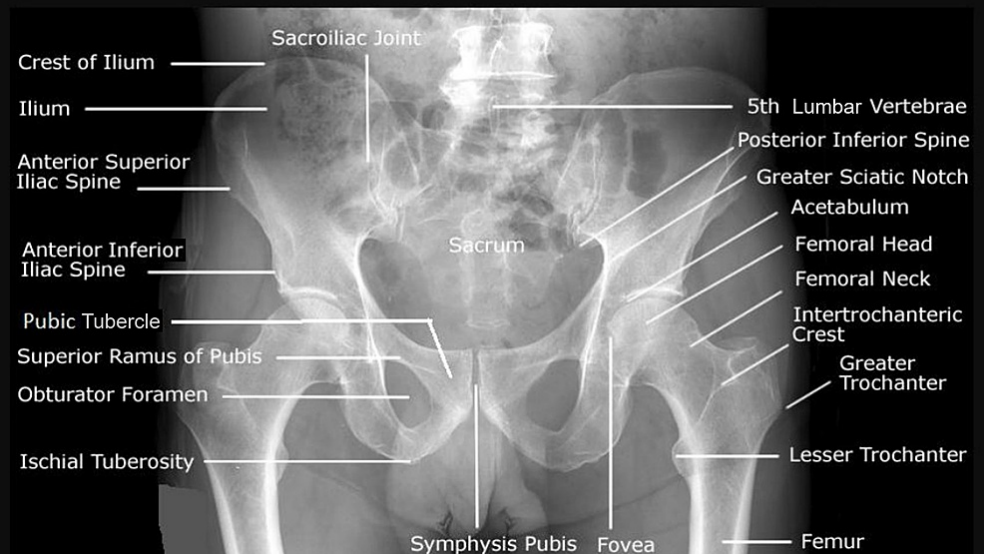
Plain Radiograph of the Pelvis with Bilateral Hip Depicting Bony Landmarks
